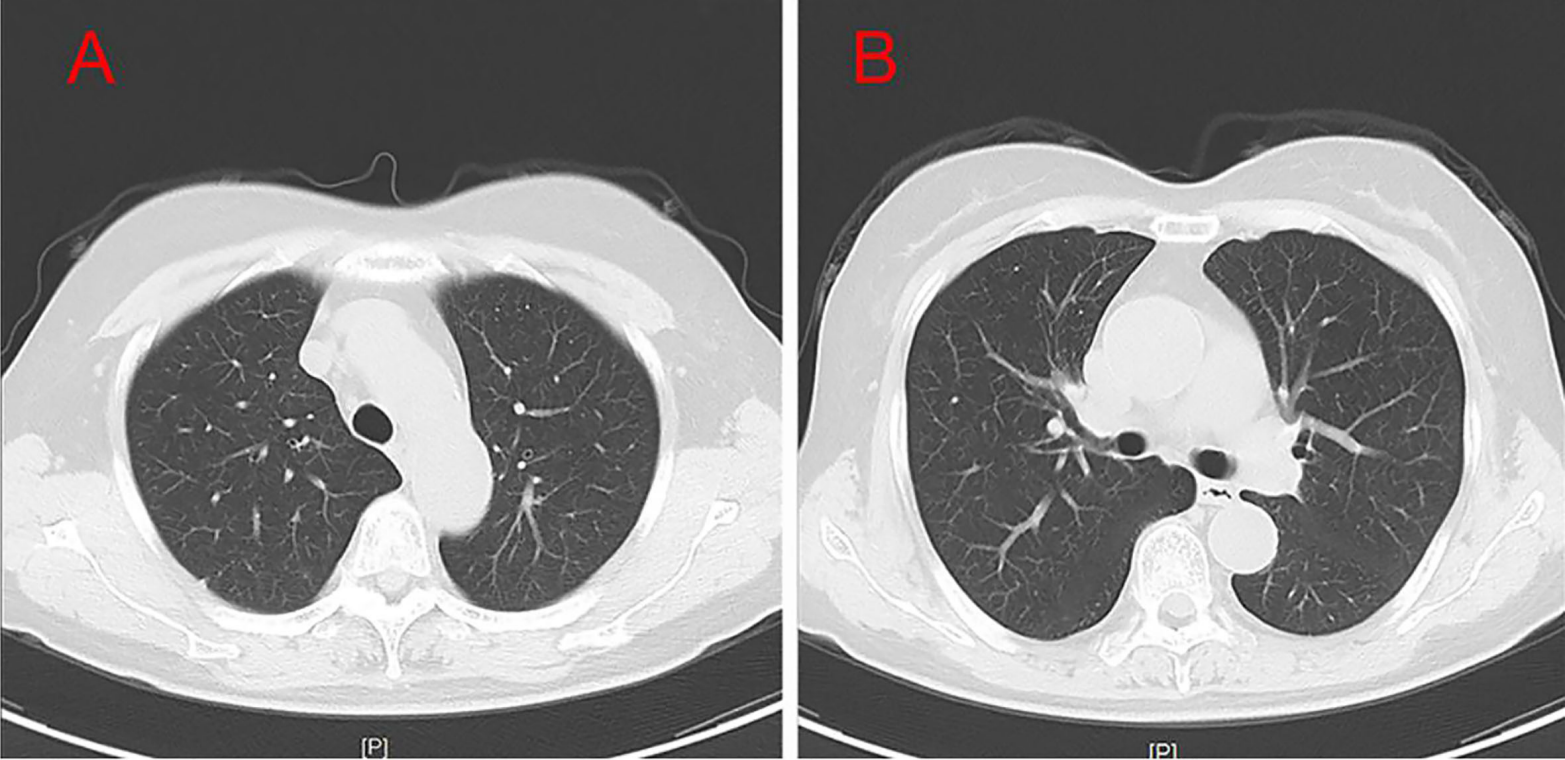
Test results of CT (A, B). Chest CT results showed that the patient had bronchitis and multiple lung nodules.
